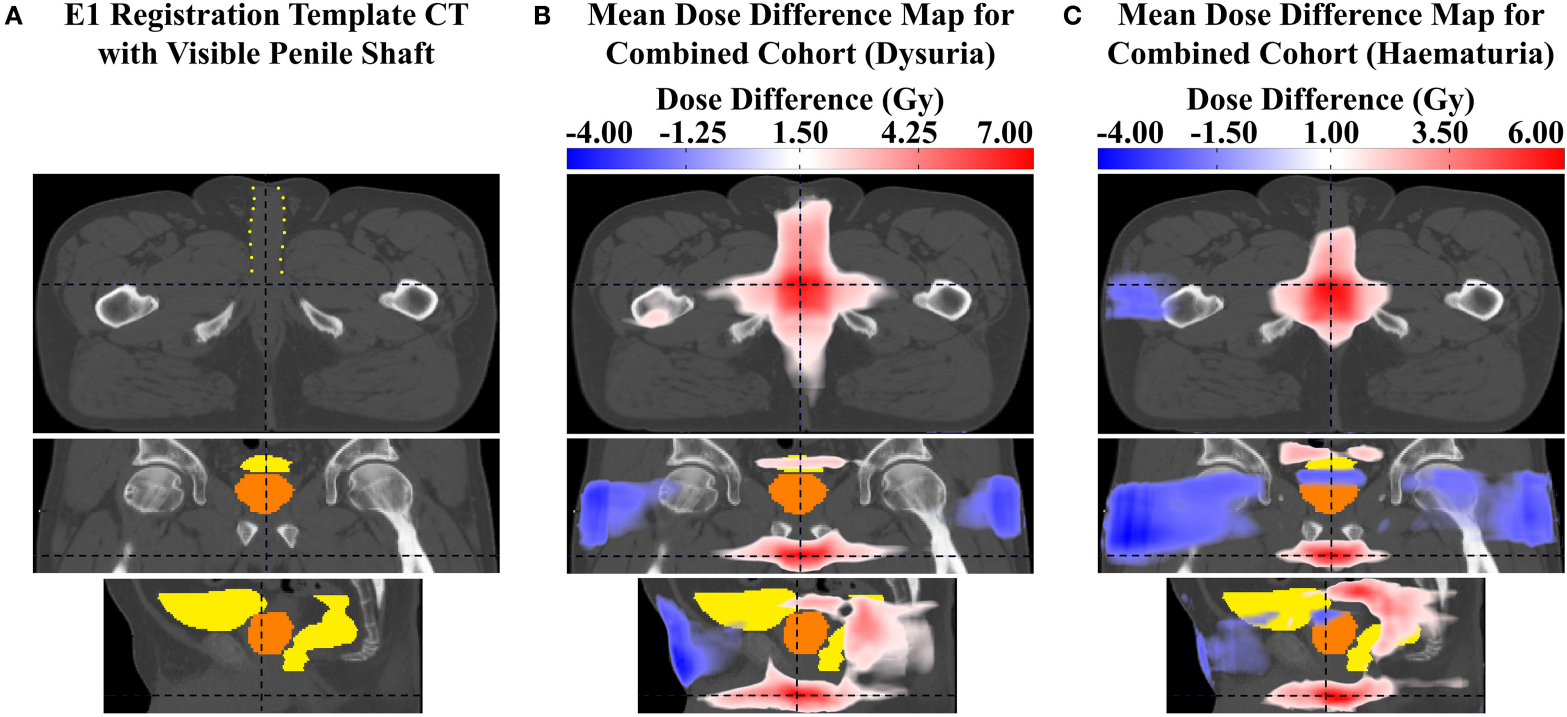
(A) slices of the T1 registration template CT image with the penile shaft (containing the spongy urethra) visible despite not being delineated, outlined in yellow dots. Mean dose difference maps between patients with and without (B) grade ≥ 2 dysuria and (C) grade ≥ 1 haematuria, on approximately the same urethral slice as the image in (A) for comparison. It is evident that maximum dose difference is most likely occurring at the urethra.
